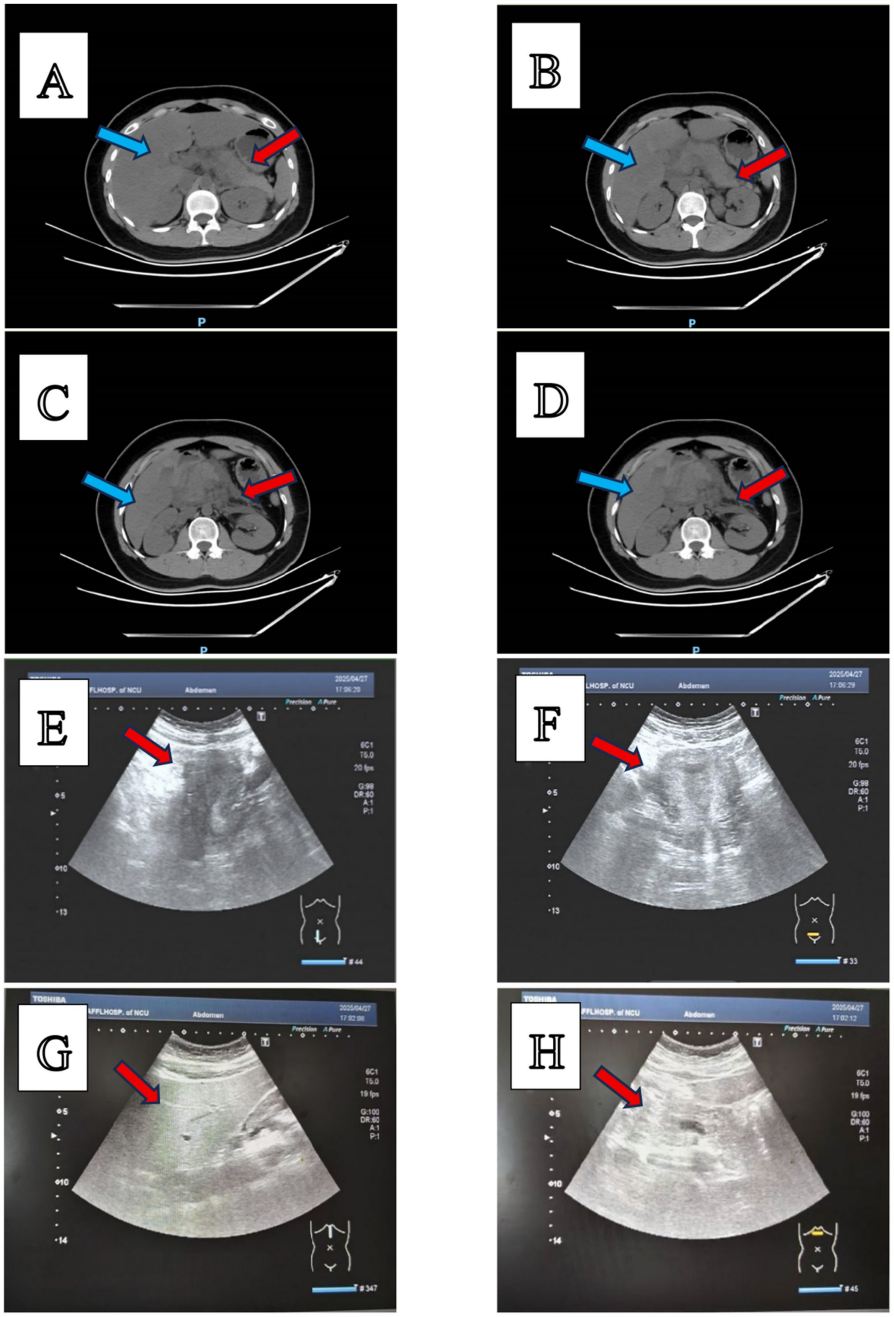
Imaging findings with parts (A–H). (A–D) Abdominal contrast-enhanced computed tomography (CT) images. The red arrows indicate the pancreas, and the blue arrows indicate the liver. CT findings include: (1) Diffusely decreased hepatic parenchymal density with indistinct visualization of intrahepatic blood vessels; and (2) Mild enlargement of the pancreas, accompanied by heterogeneous reduction in parenchymal density, blurred margins, and scattered patchy hypodense lesions in the peripancreatic region. (E,F) Gynecological color Doppler ultrasound images. The arrows point at the uterus, in which no abnormal echoes were detected. (G,H) Abdominal ultrasound images. The arrows indicate the liver parenchyma, which exhibits diffusely increased fine-grained echogenicity; the hepatic capsule remains smooth.
